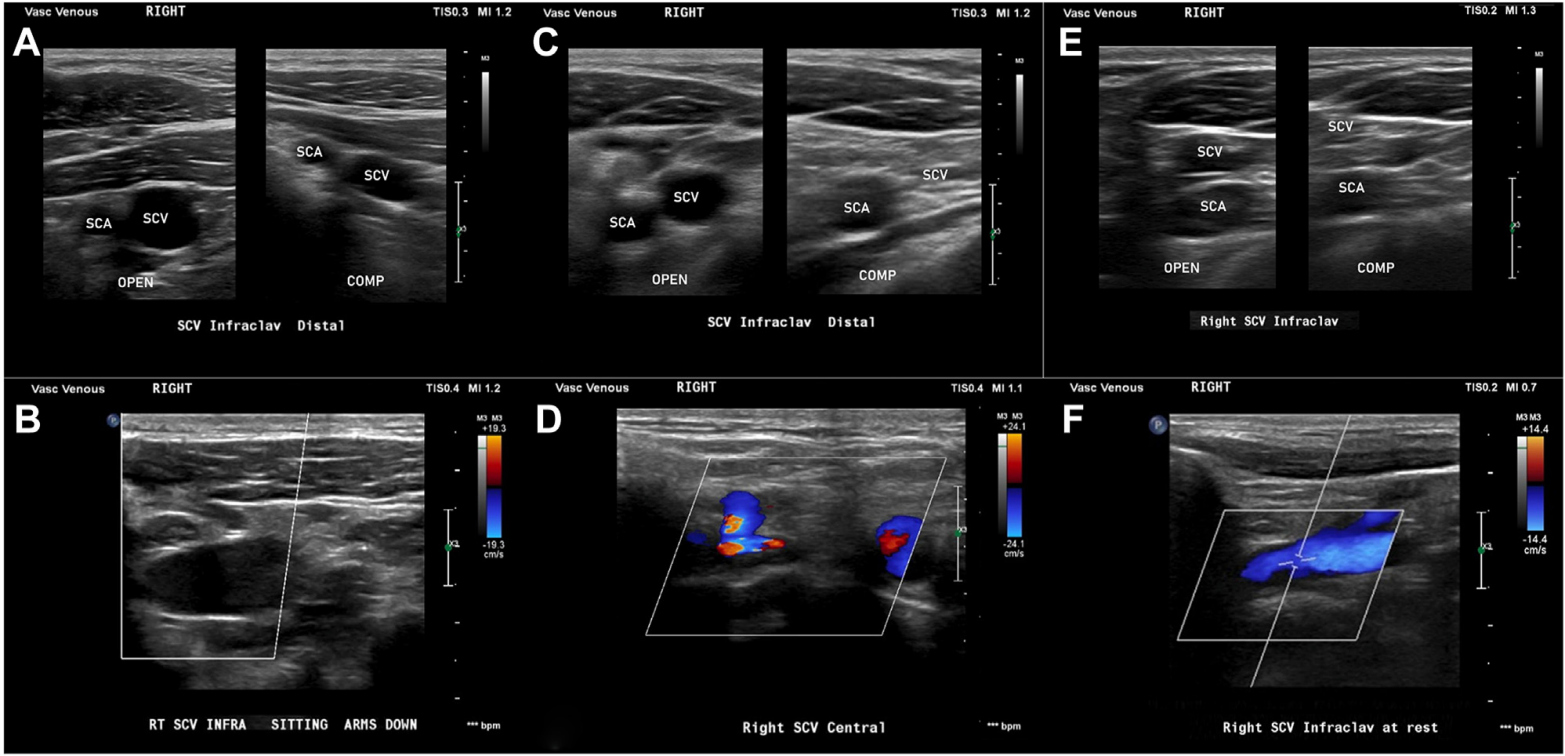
Comparison of ultrasound scans in a typical case. Before robotic first rib resection: (A) noncompressible subclavian vein (SCV) with (B) complete cessation of flow. After robotic first rib resection: (C) compressible SCV with (D) persistent stenosis in 1 SCV segment. After angioplasty: (E) compressible SCV with (F) patent SCV. (COMP, compressed; SCA, subclavian artery.)
